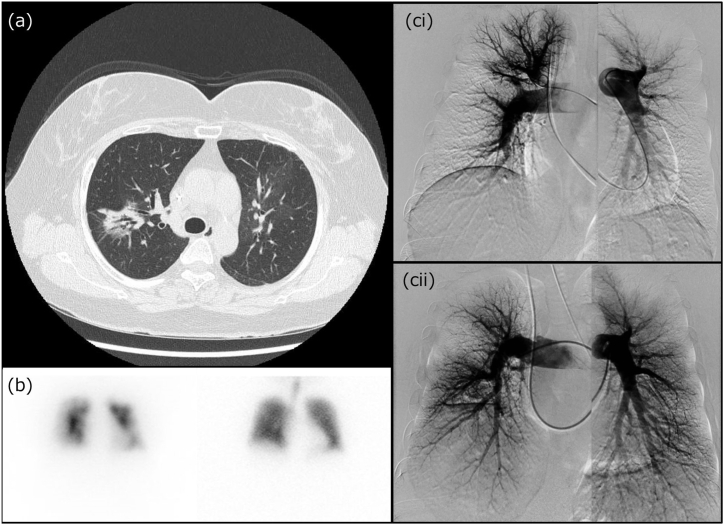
Radiological images (a) Chest computed tomography showing a part-solid ground-glass opacity with a long diameter of 3 cm in the upper lobe of the right lung (arrow), which turned out to be papillary adenocarcinoma. (b) Pulmonary perfusion scintigraphy presenting multiple defects bilaterally, whereas ventilation scintigraphy shows no defects. (ci) Pulmonary angiography performed at the time of diagnosis, showing weak distal perfusion on both sides. (cii) Pulmonary angiography performed after the third BPA session and lobectomy of the right upper lobe, showing an improvement in blood flow.
